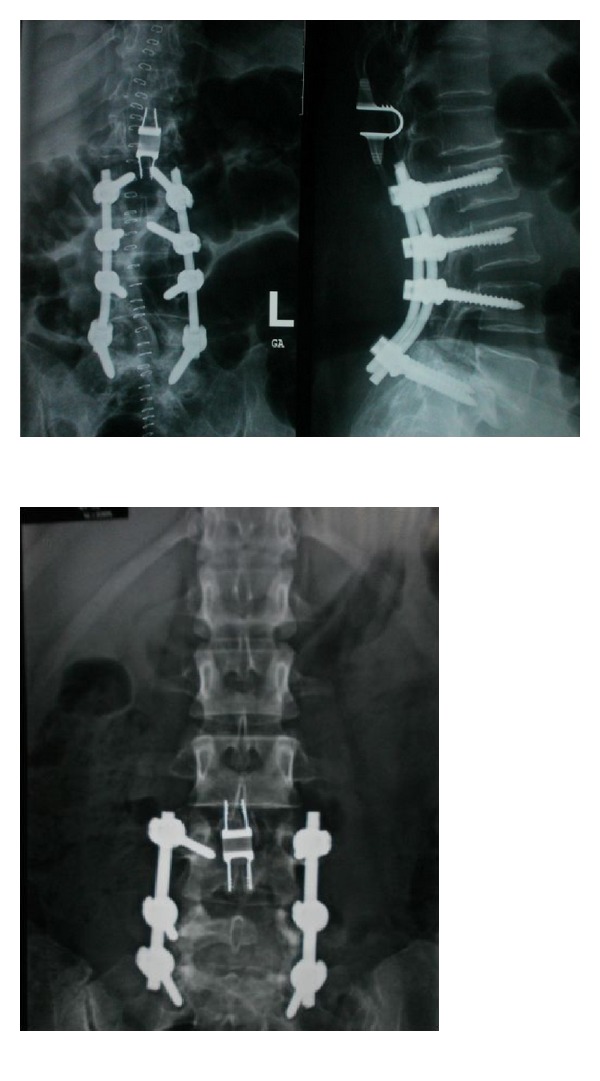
Postoperative spine X-ray, on the right, showing multiple level fusions with ISD 1 level skipping the adjacent segment. On the left, ISD used at the level adjacent to the rigid fusion.
